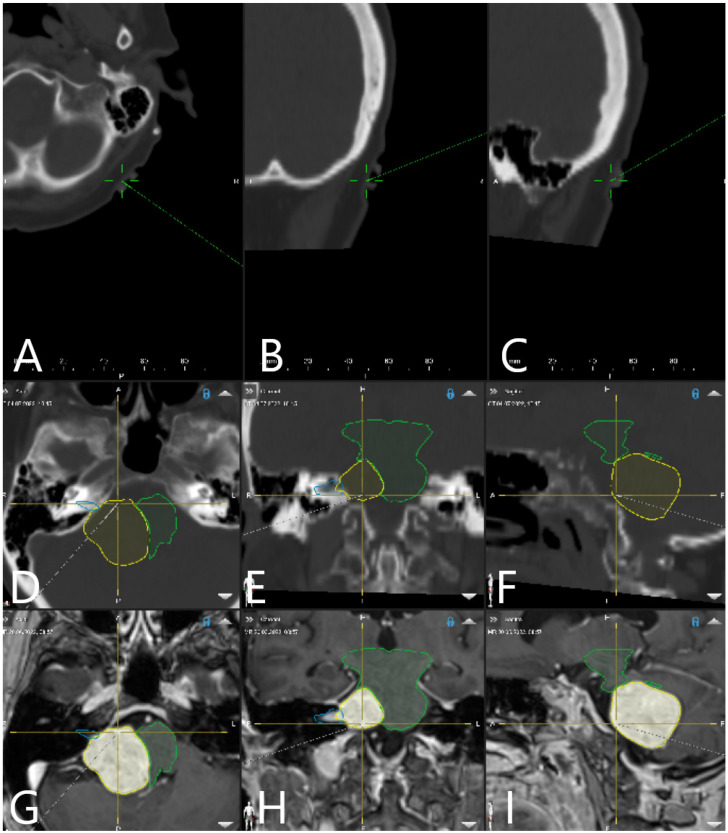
Patient No. 34. Preoperative MRI with segmented objects of interest was fused with preoperative navigation-CT with fiducials. Registration accuracy check on skin fiducial in (A) axial, (B) coronar and (C) sagittal view. Segmented objects of interest (tumor outline in yellow and brain stem in green) are presented in (D) axial, (E) coronar and (F) sagittal CT, as well as in (G) axial, (H) coronar and (I) sagittal T1-weighted post-contrast MRI.
